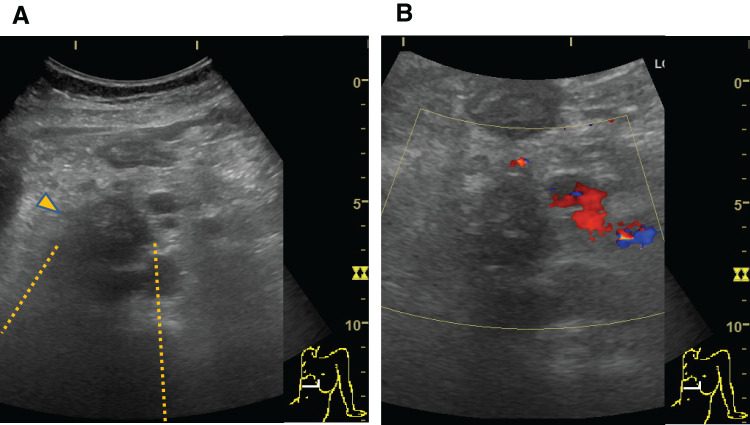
Abdominal ultrasonography images. (A) Abdominal ultrasonography image revealing a heterogeneous hypoechoic mass in the groove area. High-echo structures within the mass are accompanied by acoustic shadowing. Arrowhead: mass. Dotted line: acoustic shadowing. (B) Color Doppler examination image showing no Doppler signals within the mass.
